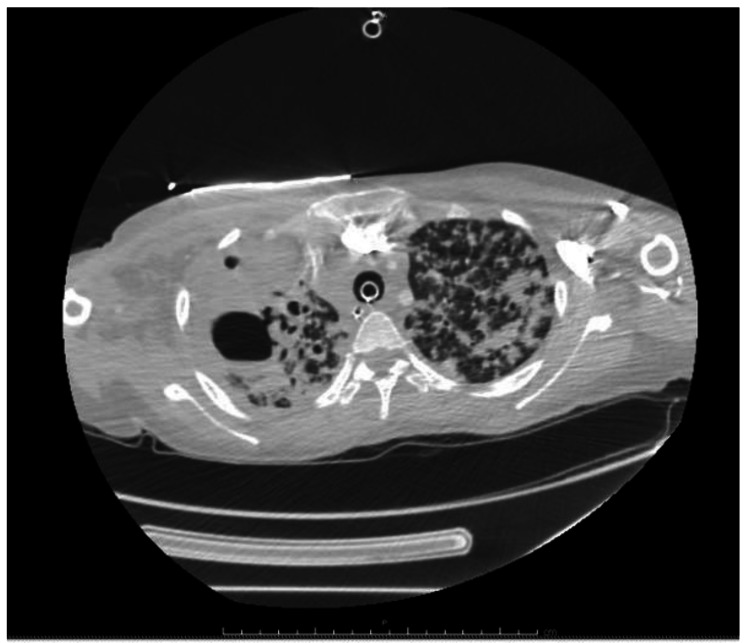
Computed tomography angiography (CTA): Extensive pulmonary opacities and right upper lobe cavitary lesion in a distribution most worrisome for post primary TB
